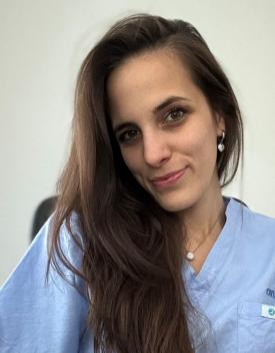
Henriette Mészáros is a cardiologist in training at the Heart and Vascular Centre of Semmelweis University in Budapest, Hungary with a special interest in echocardiography and atrial fibrillation. Her primary focus lies in stroke prevention and the cardiac diagnostic workup following ischaemic stroke, which serves as the basis for this publication. Through her research and clinical practice, she aims to contribute to advancements in cardiovascular imaging and stroke management. This work reflects her commitment to improving diagnostic precision and outcomes for patients with cerebrovascular and concomitant cardiovascular conditions.
